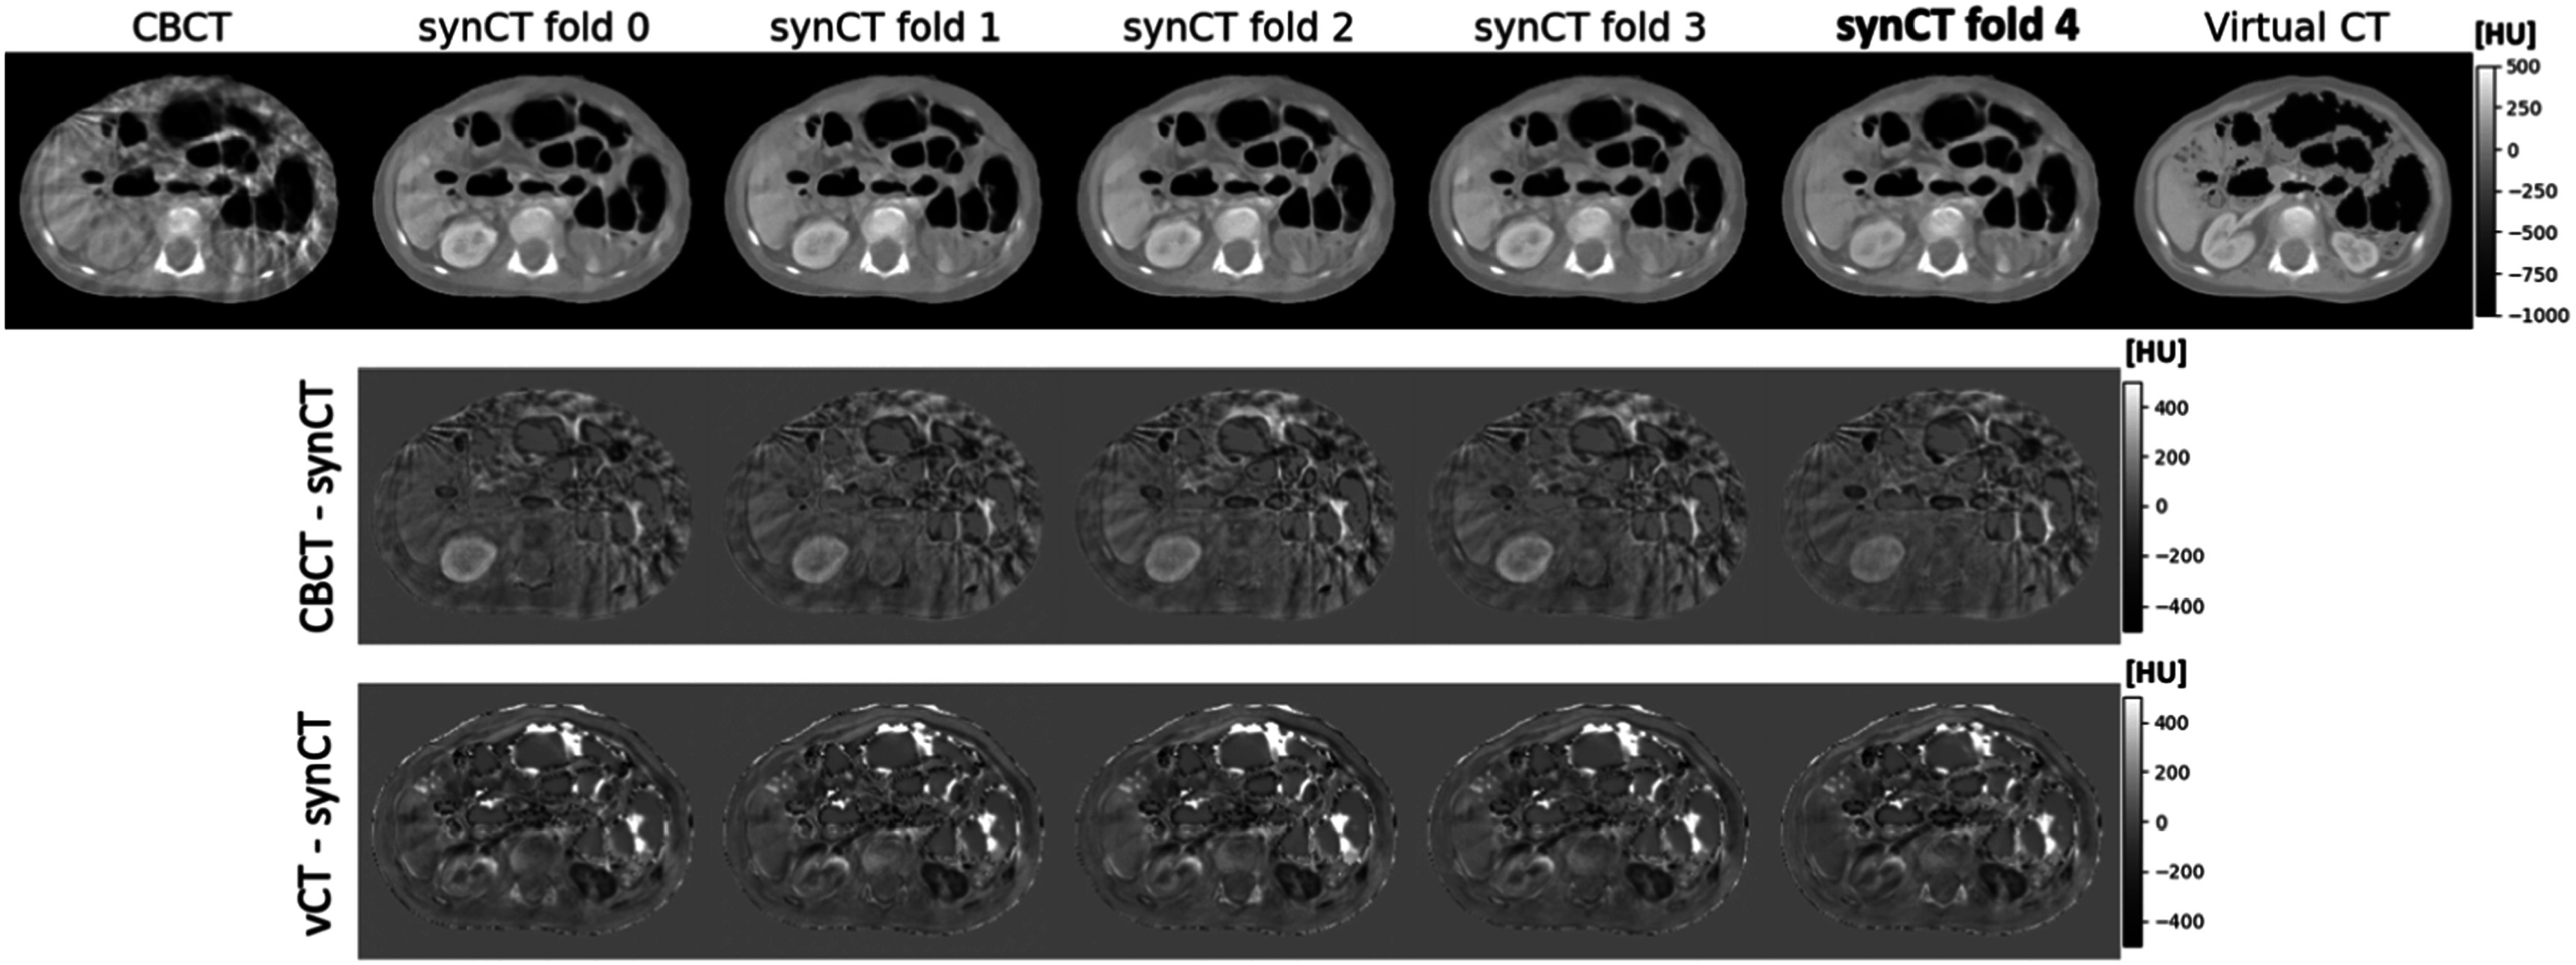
Comparison of synthetic CTs between different folds when 5-fold cross validation was applied. The fold where the slice was part of the validation subset is shown in bold (fold 4). For the remaining folds the slice was used for training. No significant visual differences in anatomy were observed. The two lower rows show the difference images between the output synthetic CTsand the CBCT (top) and virtual CT (bottom).
